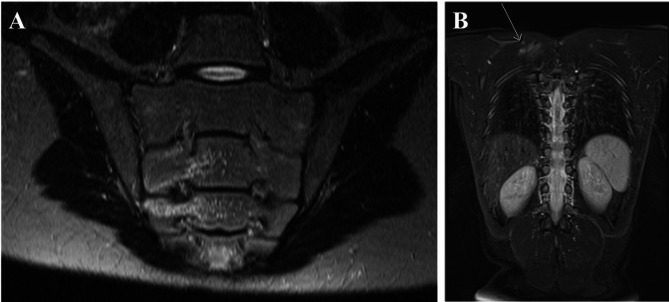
Examples of injuries found in the whole-body MRI after polytrauma
